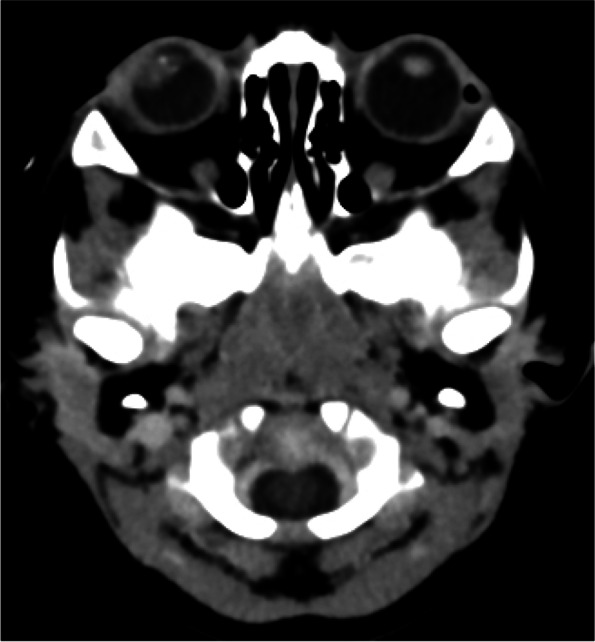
CT head axial non-contrast demonstrates right microphthalmia with a soft tissue mass associated with the lens, with a focus of calcification
